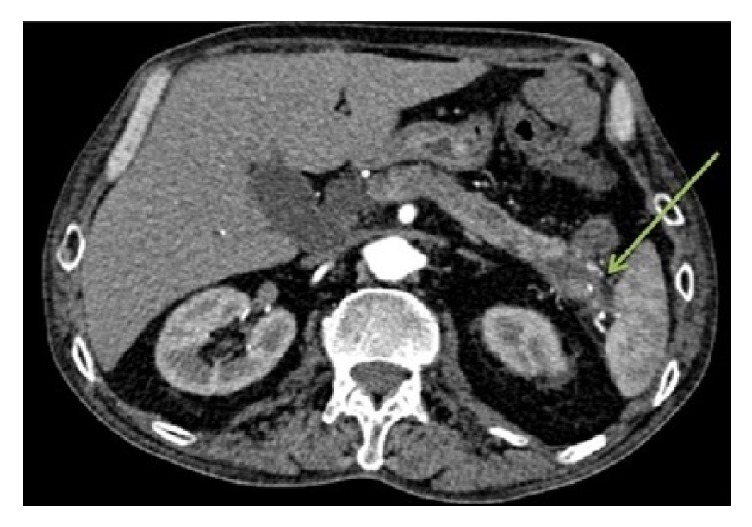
CTA showing a pancreatic tail lesion, with a focus of fluid and interval diffuse progression of colonic wall thickening. The pancreatic tail was inseparable from the colon, with a possible connection between the colon and the pancreas. Green arrow denotes possible connection between the colon and pancreas.
